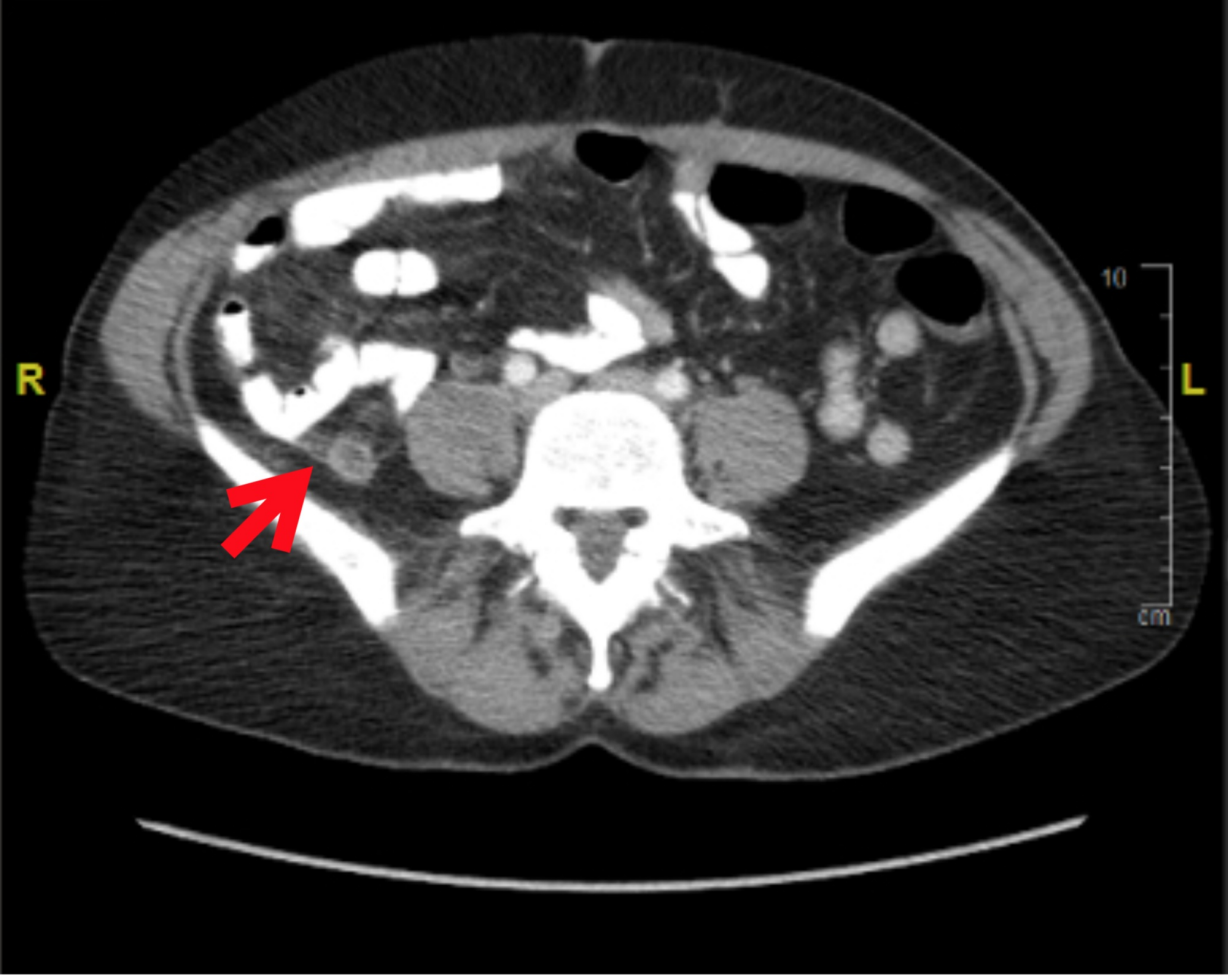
Axial CT scan demonstrating appendicitis CT: computed tomography
